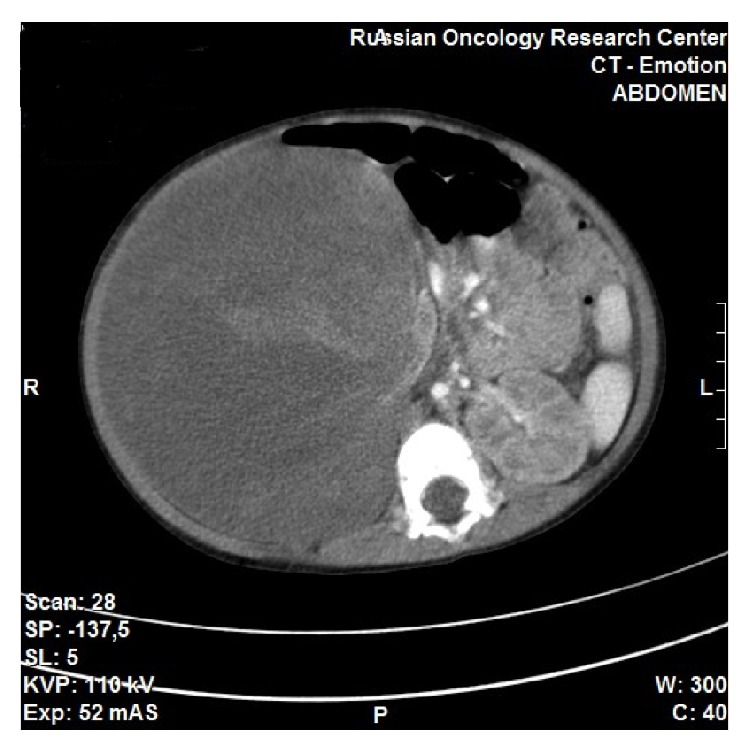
Preoperative abdominal computed tomography: right kidney subtotally replaced by tumor.
